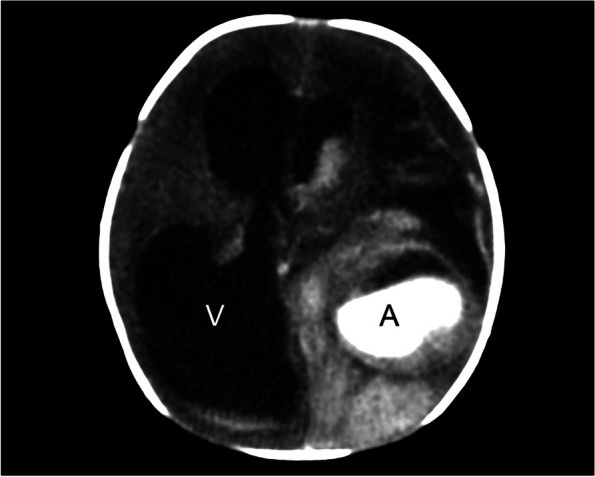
Neonatal CT brain with contrast on day 1 after birth shows marked ventriculomegaly (V) and bright hyperechoic area representing active flow in the aneurysm (A), surrounded by hyperechoic area of hemorrhage
